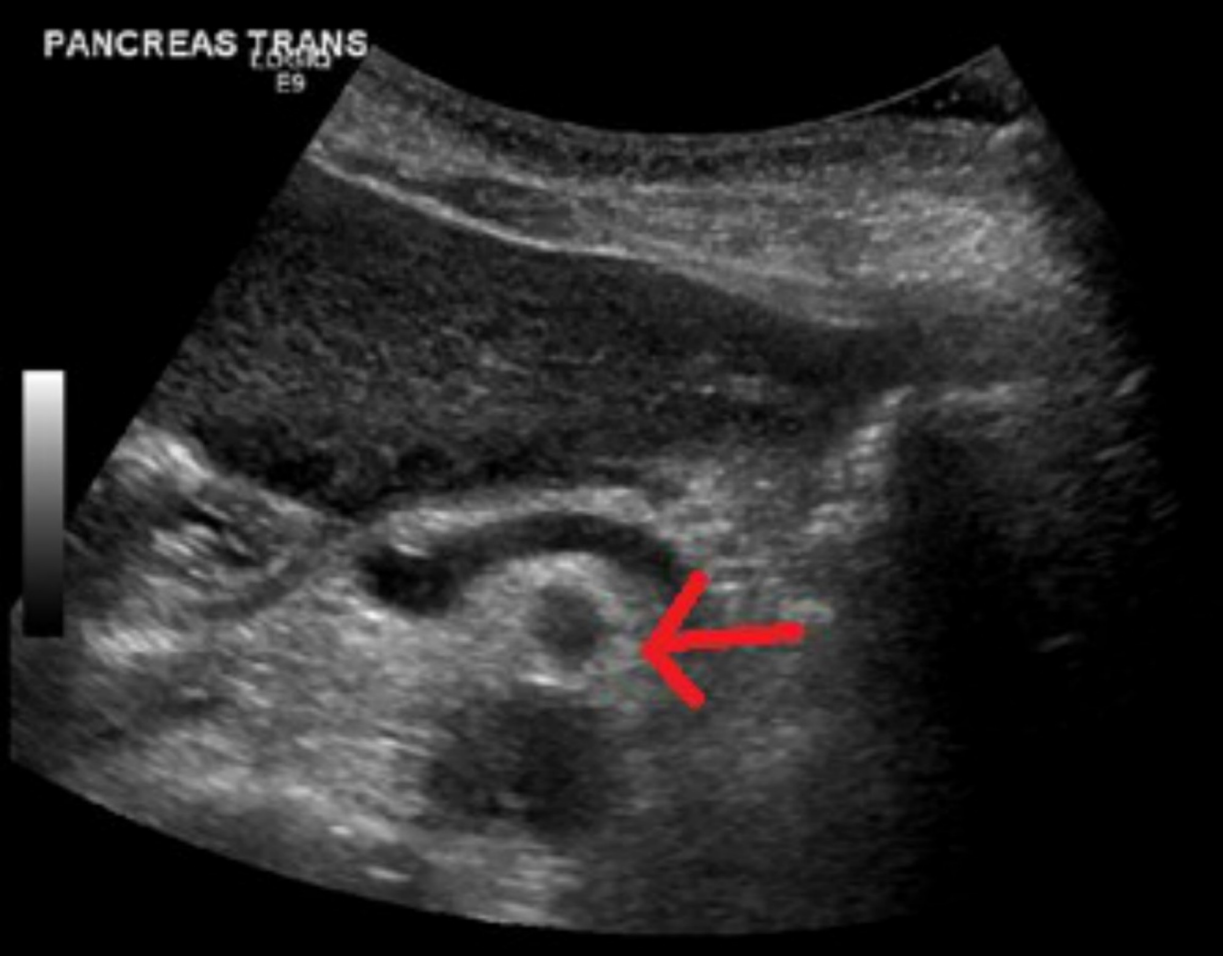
Ultrasound of abdomen; heterogenous hypoechoic mass in subcutaneous tissues of left lower quadrant.
